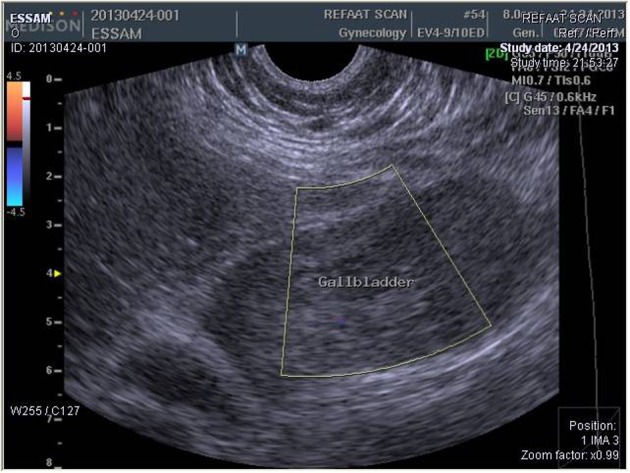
Abdominal ultrasound with color flow doppler showing huge gall bladder hematoma filling the whole lumen with absence of color flow waves.
